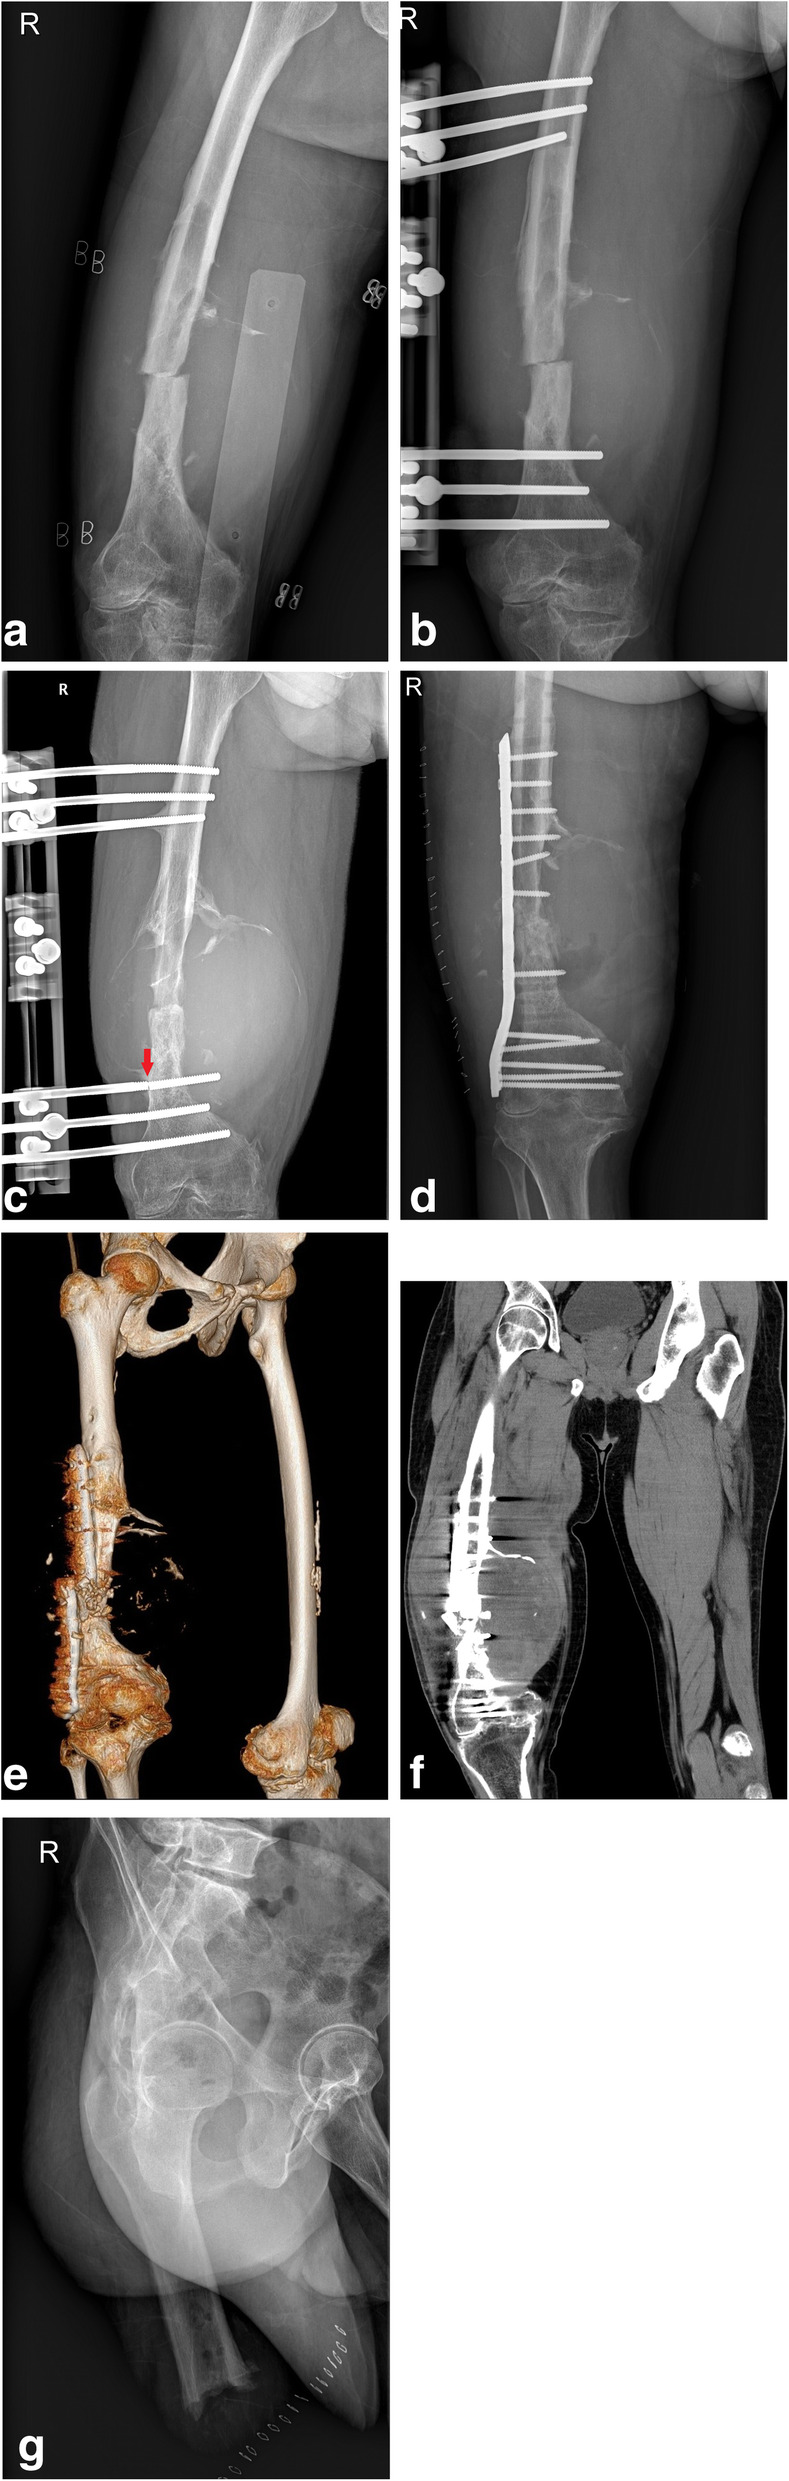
Surgical treatment with CREF to a 46-year-old man (case 2) with a right femoral shaft HPT-related fracture. a, b Preoperative and postoperative radiograph of CREF. c Bone nonunion combined with fixator failure (red arrow) 13 months later. d ORIF performed and HPT excision 14 months later. e, f HPT recurrence and plate broken 6 months after ORIF. g Amputation performed finally
